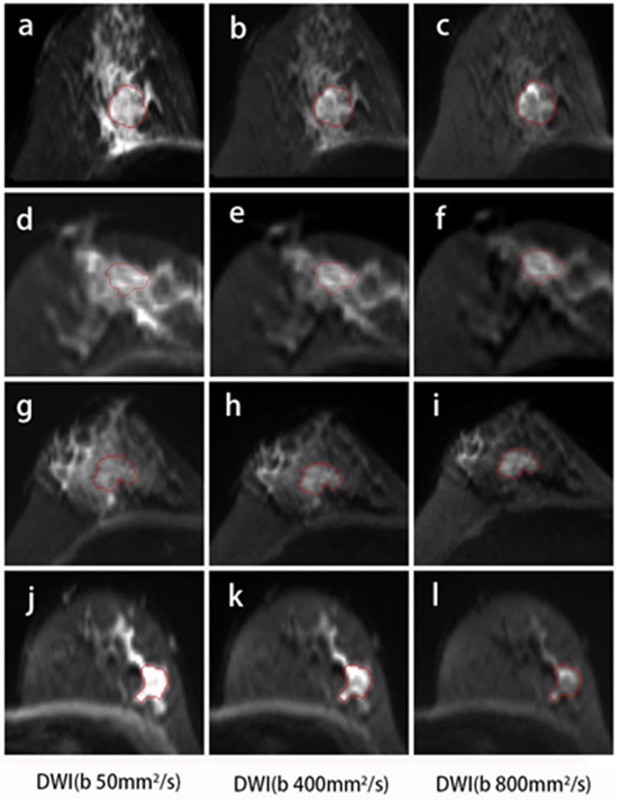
DWI images for the four subtypes of breast cancer. A 46-year-old female with Luminal A breast cancer (A–C); a 60-year-old female with Luminal B breast cancer (D–F); a 47-year-old female with HER2-positive breast cancer (G–I); and a 57-year-old female with TN breast cancer (J–L). The small dependence emphasis of Kendall-tau-b for DWI_sequential_ for Luminal A, Luminal B, HER2-positive, and TN breast cancers were −0.915, 0.358, 0.915, and 0.299, respectively.
